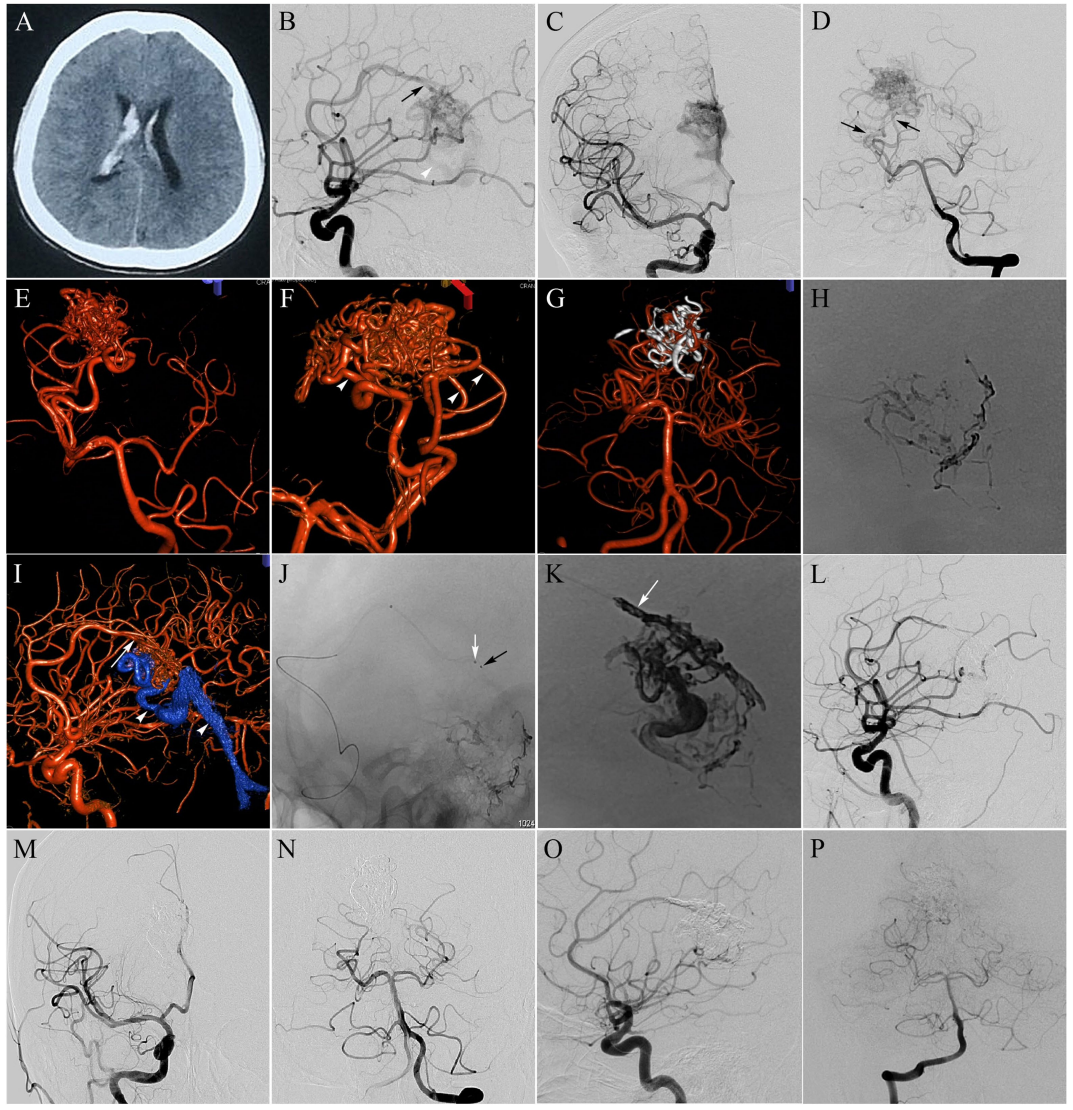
Callosal AVM, Spetzler-Matin grade II. The patient presented with severe headache and meningeal irritation syndrome. (A) Axial CT scan at presentation, revealing intraventricular hemorrhage; (B–D) Selective right internal carotid artery (ICA) injection [anteroposterior (B) and lateral (C) views] and selective left vertebral artery (VA) injection [anteroposterior (D) view] DSA indicating a 2.8-cm AVM nidus, supplied by a hypertrophied pericallosal artery originating from the right anterior cerebral artery (ACA; black arrow in B), as well as 2 main feeders from the right posterior cerebral artery (PCA; black arrows in D), and drained by the same venous outlet (white arrowheads in B and I), into the straight sinus; (E,F) Three-dimensional rotational angiography better delineated the arterial feeders from the PCA. Three feeding arteries were selected for preliminary Onyx embolization (white arrowheads in F). The goal of this embolization was nidus volume reduction; (G) Onyx cast after completing preliminary embolization following 3D reconstruction; (H) Single-shot DSA revealing Onyx cast after preliminary embolization; (I) Three-dimensional rotational angiography showing enlarged pericallosal artery (white arrow) feeding the nidus; (J) Single shot showing the markers of two microcatheter tips of the Marathon (black arrow) and the SL-10 (white arrow) inside the pericallosal artery; (K) A plug comprising coils and glue was generated to avoid reflux (white arrow). Single-shot DSA revealing Onyx cast after procedure completion; (L–N) Selective right ICA and left VA injection DSA performed immediately postoperatively showing complete nidus exclusion; (O,P) At 12-month follow-up, angiography showed persistent total occlusion.
